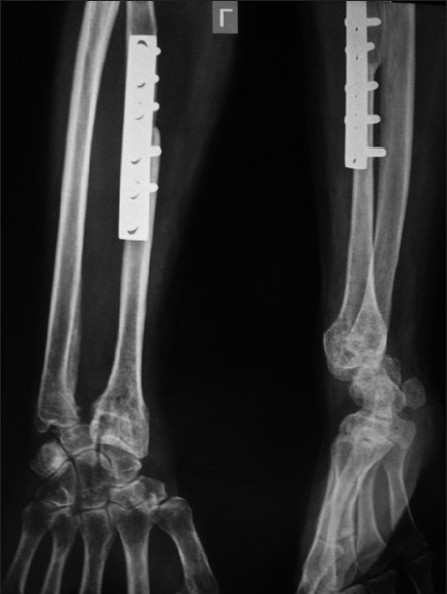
X-ray – Anteroposterior and lateral views showing moderate subluxation of fibulo-carpal joint with ulno-fibula diastasis
